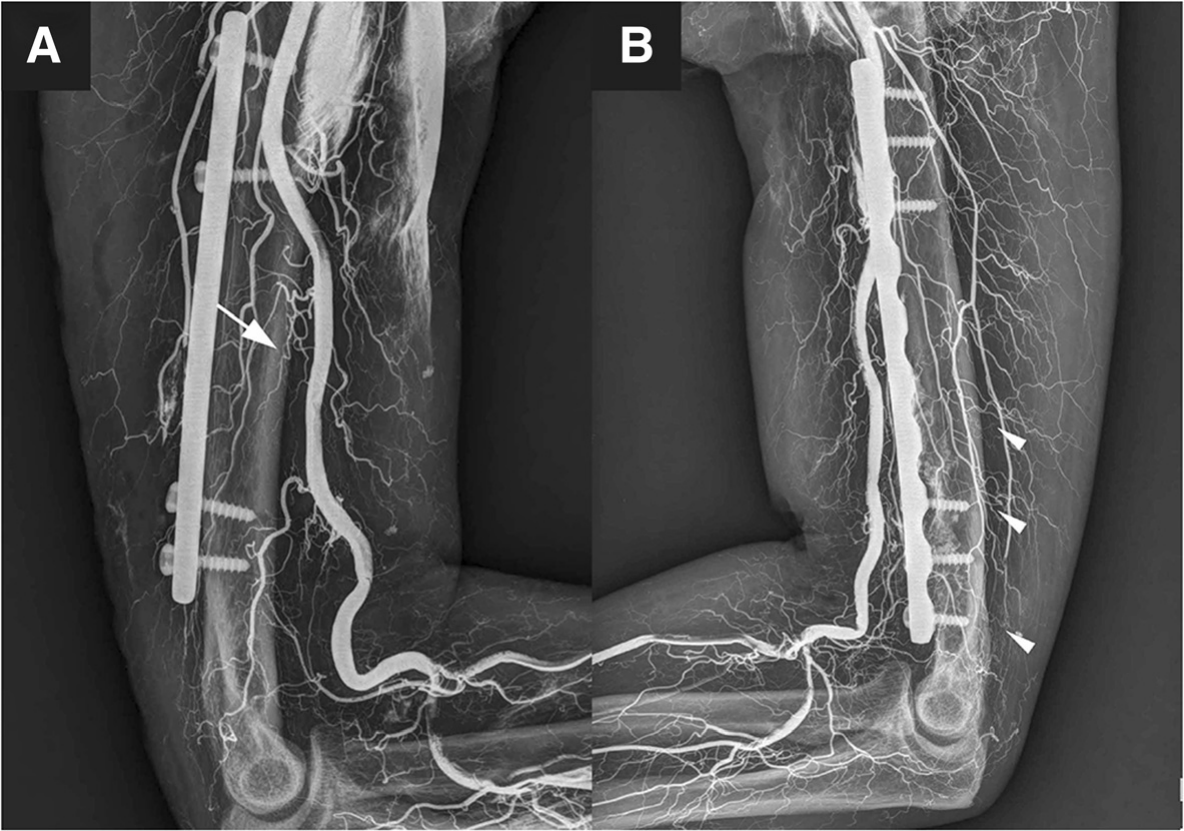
Radiograph images of vascular perfusion. a shows the main nutrient artery (denoted by *arrow*) of the humeral shaft, b shows the accessory nutrient arteries (denoted by *arrow heads*) of the humeral shaft
